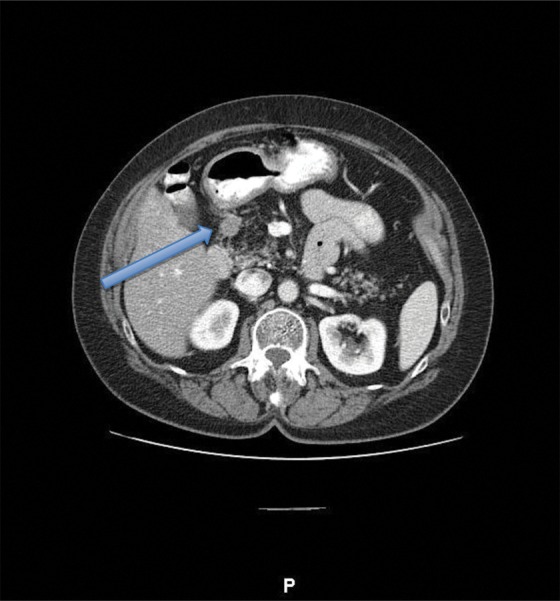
Computed tomography scan showing a 2 cm mass (arrow) in the pancreaticoduodenal groove near D1–D2.
